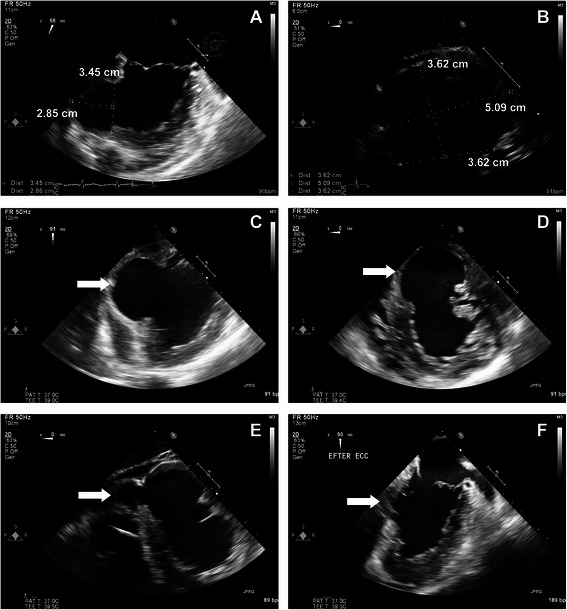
Peri-and postoperative TEE. Perioperative transesophageal echocardiogram demonstrates that the cavity is almost as large as that of the LV, and probably has increased in size from the previous TTE (a–d), white arrow points to the pseudoaneurysm. The cavity dissects beneath the septum and under the right ventricle (e), white arrow points to the part beneath the RV. TEE at the end of surgery shows that the cavity is largely obliterated (f), the white arrow points to where the ostium was
